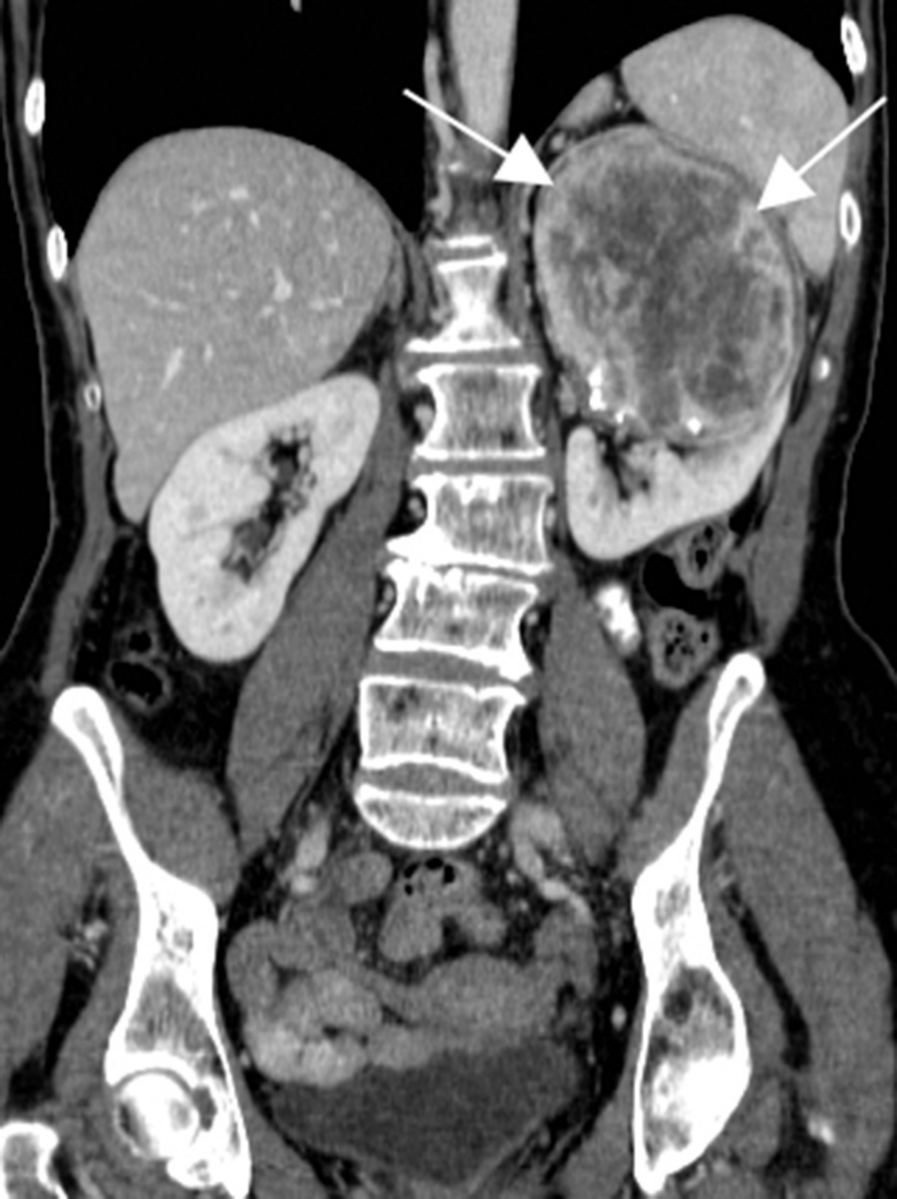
CT appearance of the SFT. A coronal contrast-enhanced CT image demonstrates a heterogeneous peripherally enhancing mass in the upper pole of the left kidney (white arrows).
